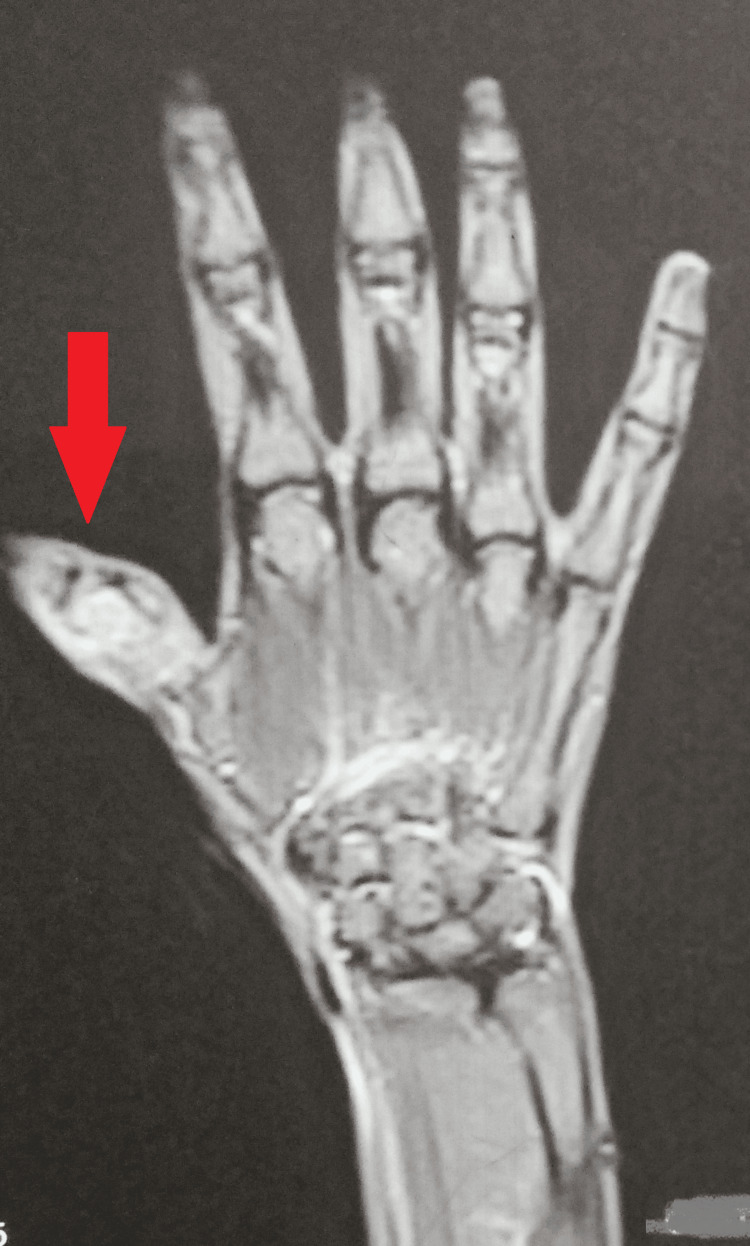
Magnetic resonance imaging of the left wrist showing significant involvement of the left thumb.
